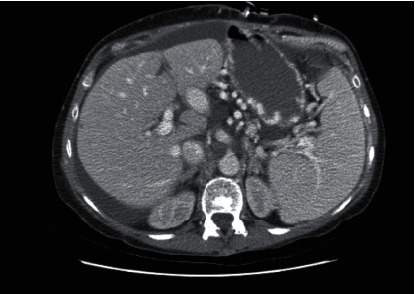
Posttransplant CT scan demonstrating large varices, splenomegaly, and ascites.
